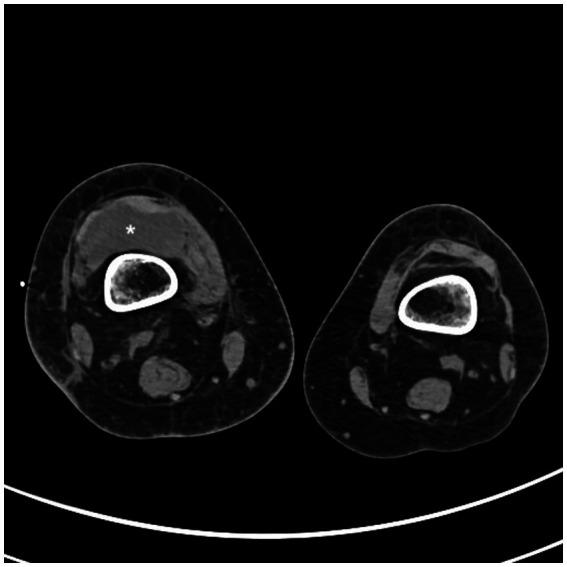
CT scan of the knees showing a voluminous intra-articular right effusion (*).
